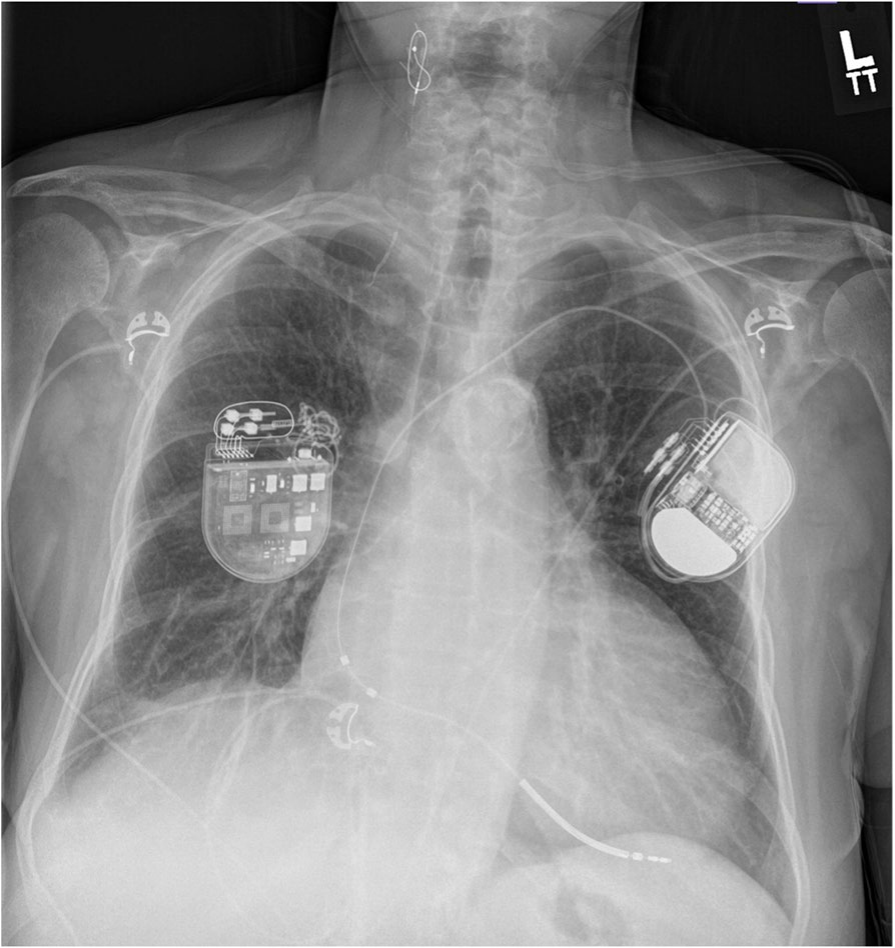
Chest X-ray. Eight months post-implantation of Barostim NEO device with fractured lead. The proximal lead demonstrates significant coiling, twisting, and retraction consistent with Twiddler’s syndrome
